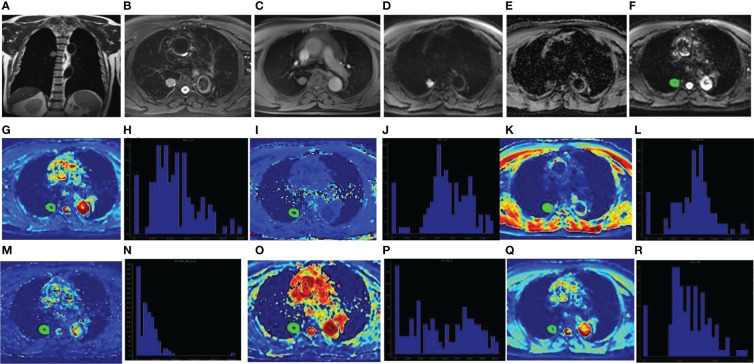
Male, 67 years old, the dorsal segment of right lower lobe showed solid SPN with a length of about 22mm. The pathological findings were adenocarcinoma. Coronal T2WI showed Slightly higher signal (A); Axial T2WI and fat suppression sequence showed uneven slightly higher signal with rough edges and adjacent pleural depression (B); Axial T1WI showed isosignal (C); DWI showed high signal (D) and ADC value decreased (E). The ROI was delineated layer by layer on the original image (F) in the DKI model, and then a VOI was synthesized and copied to the pseudo-color images of Dk (G), K (I), D (K), D* (M), f (O) and ADC (Q). The whole-lesion histogram parameters of Dk (H), K (J), D (L), D* (N), f (P) and ADC (R) value were calculated. D* is one of the parameters of IVIM.
